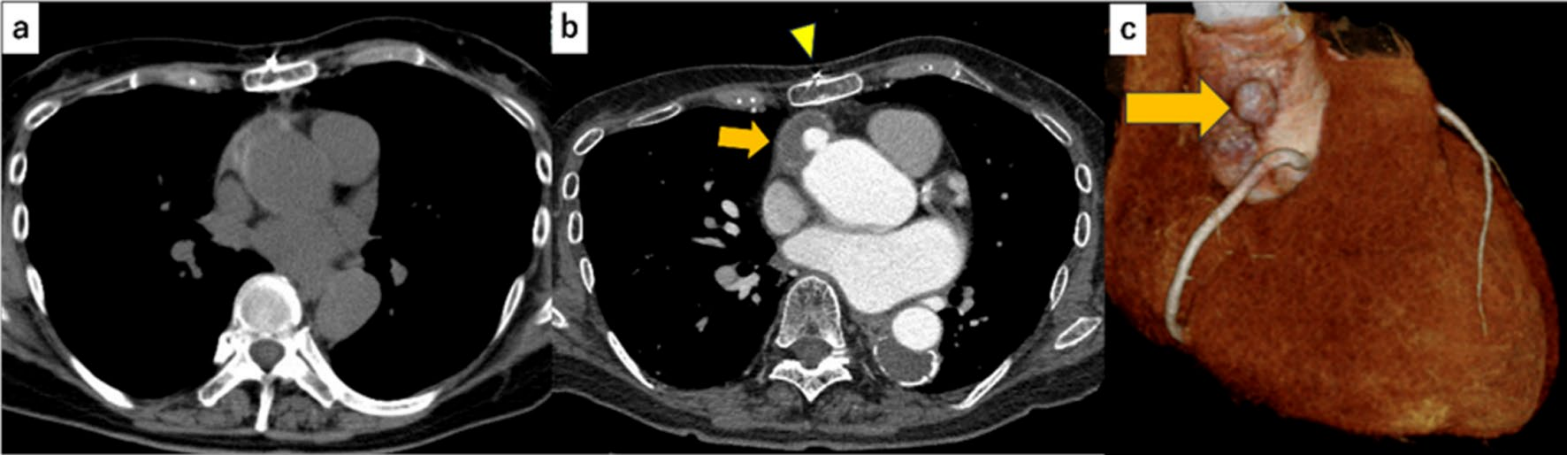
Computed tomographic images of aortic root. Pre-trauma (a) and post-trauma (b) axial computed tomographic images, and a lateral view of the Valsalva sinus in three-dimensional volume-rendered cardiac computed tomography (c). Arrows indicate pseudoaneurysm of the aortic root. An arrowhead indicates a sternal wire
